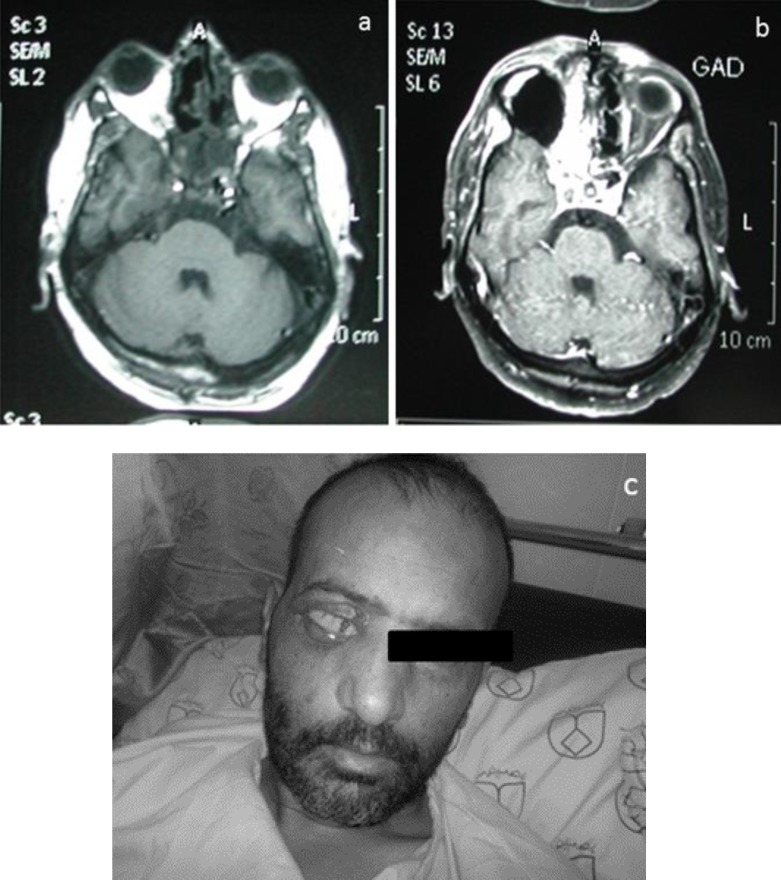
3a: In MRI study, there is evidence of enhanceable soft tissues density in right ethmoidal air cell with bone destruction and extension to right and retro-orbit and intracranial area. Opascification of right sphenoidal and ethmoidal sinus also is seen; 3b and 3c: right orbit is enucleated
